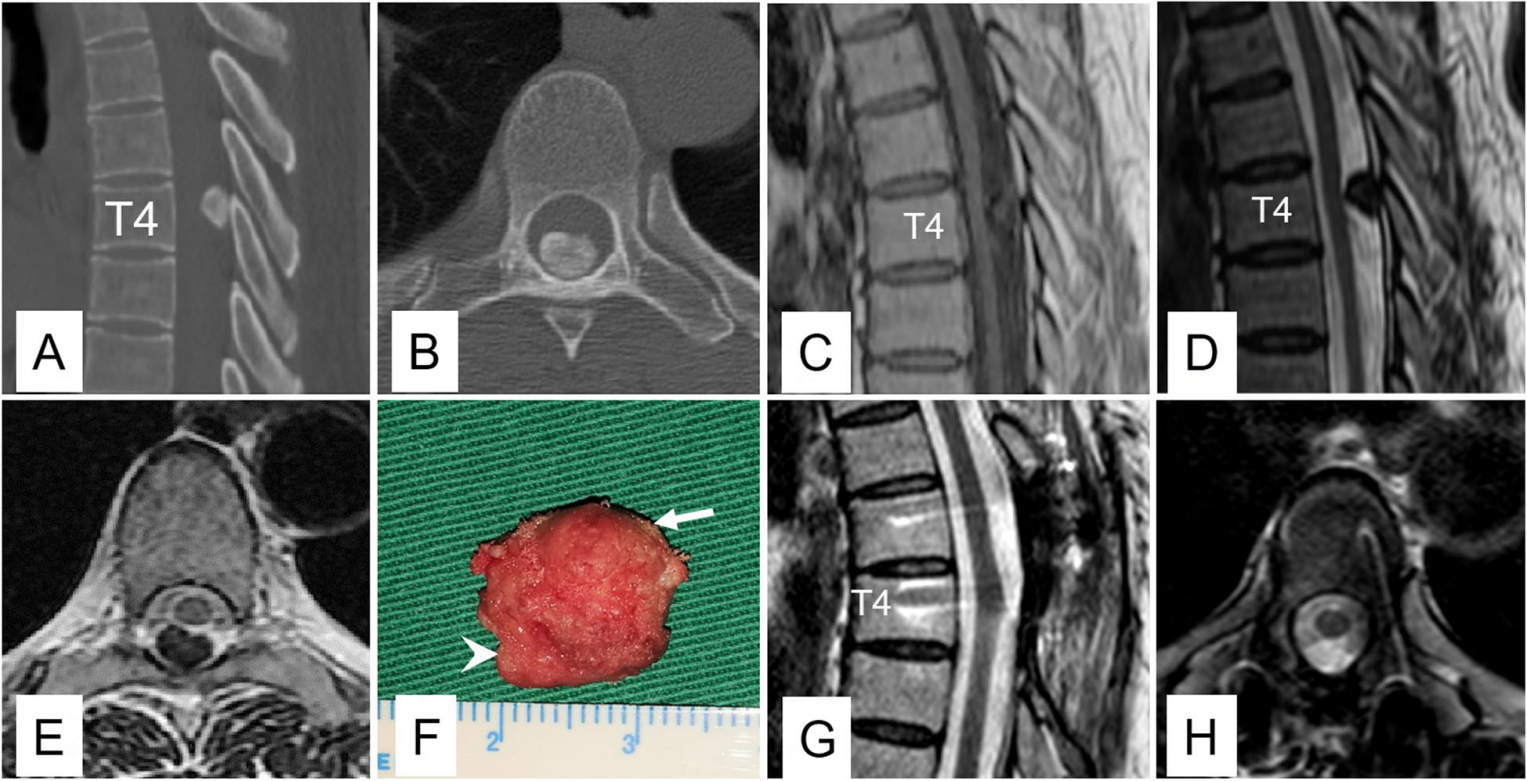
CT bone window (a–b) displayed an intraspinal tumor with high and uneven density on the dorsal side of the canal at the T4 level. Notice that there was an interspace between the neoplasm and lamina. MRI (c–e) revealed an intradural extramedullary mass, which pushed the spinal cord dorsally without obvious compression. The tumor showed hypointensity on both T1 and T2 images compared to the spinal cord. f Grossly, the tumor was a firm entity (arrow) with adhesion to the adjacent dura and arachnoid (arrowhead). Follow-up MRI images (g–h) showed no evidence of recurrence
